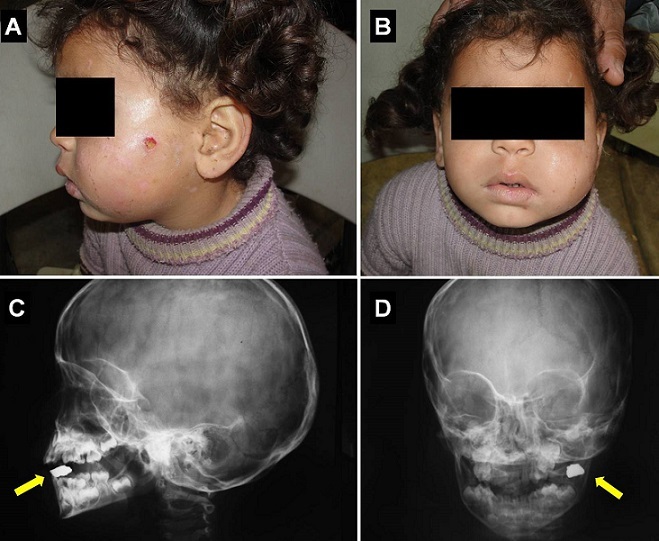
External photograph of the patient presented with left cheek swelling, facial wound discharge and difficulty to open her month completely (A, B); Plain facial radiography revealed a metallic foreign body in the left cheek (arrows) without osteolysis (C, D)
